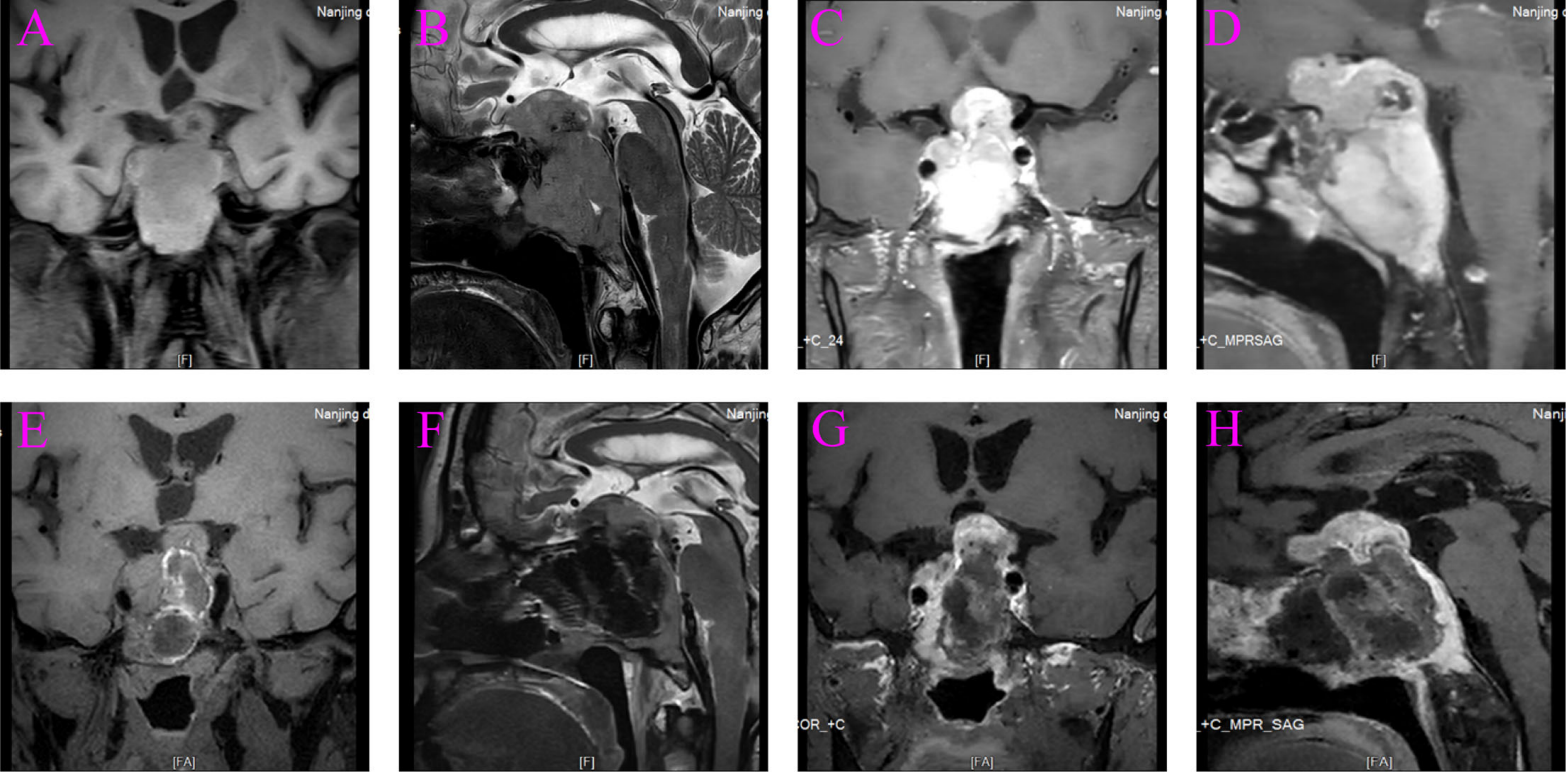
Preoperative and postoperative magnetic resonance imaging (MRI). (A) Preoperative coronal T1-weighted MRI. (B) Preoperative sagittal T2-weighted MRI. (C) Preoperative coronal T1-weighted with contrast. (D) Preoperative sagittal T1-weighted with contrast. (E) Postoperative coronal T1-weighted MRI. (F) Postoperative sagittal T2-weighted MRI. (G) Postoperative coronal T1-weighted with contrast. (H) Postoperative sagittal T1-weighted with contrast.
